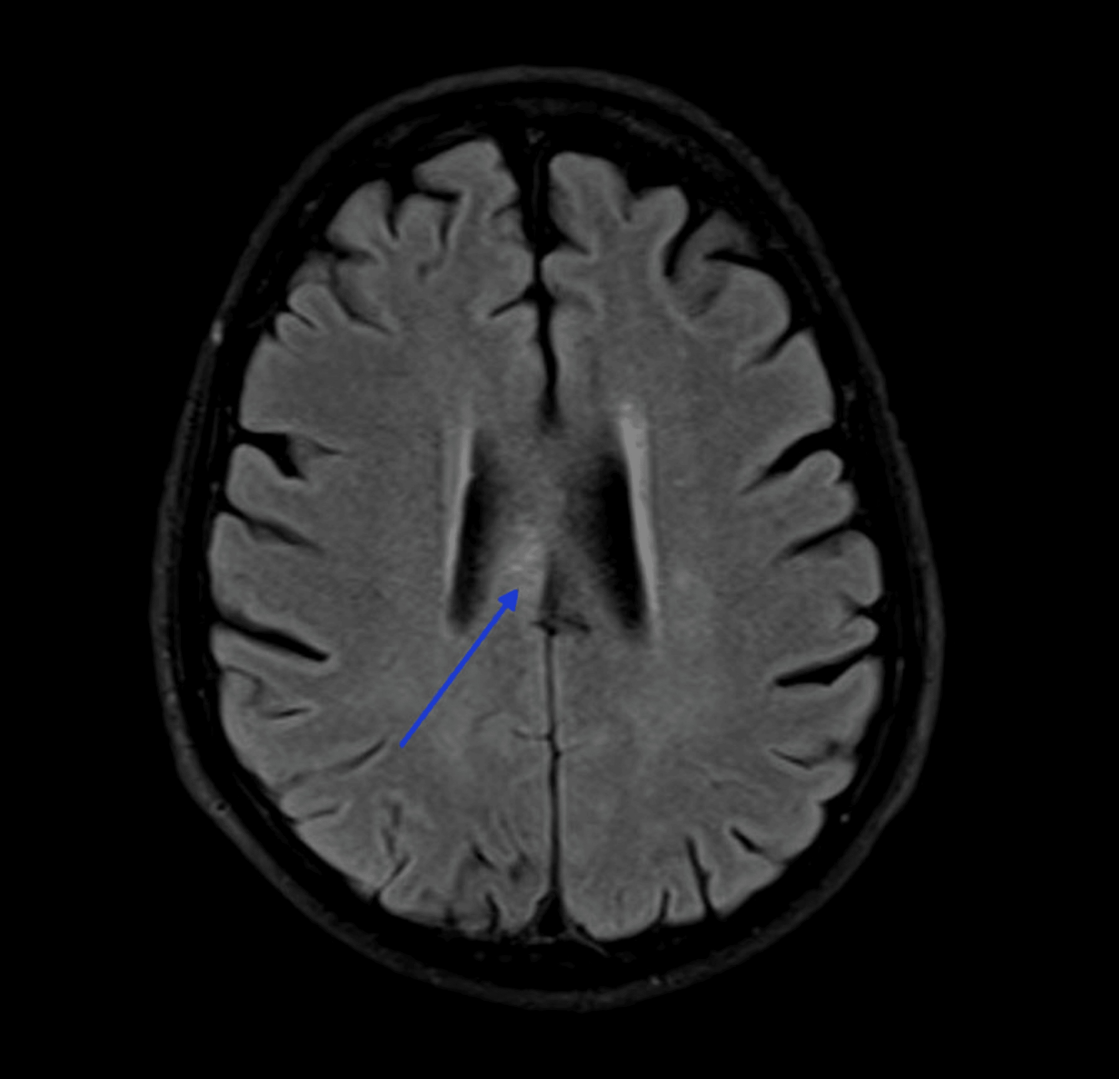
MRI brain, axial view, FLAIR sequence, showing hyper-intensity in the right body of the corpus callosum. FLAIR: fluid-attenuated inversion recovery.
